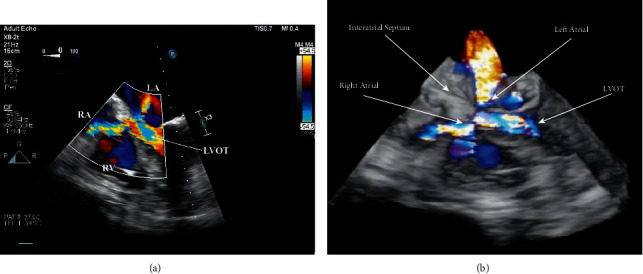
Postoperative day 2 TEE demonstrating two distinct regurgitant jets by color flow Doppler (CFD) with a common origin in the LVOT communicating with both the RA and LA. (a) 2D CFD of the mid-esophageal four-chamber view that has been cropped and (b) 3D CFD full-volume reconstruction (Philips X8-2t probe, Cambridge, MA).
